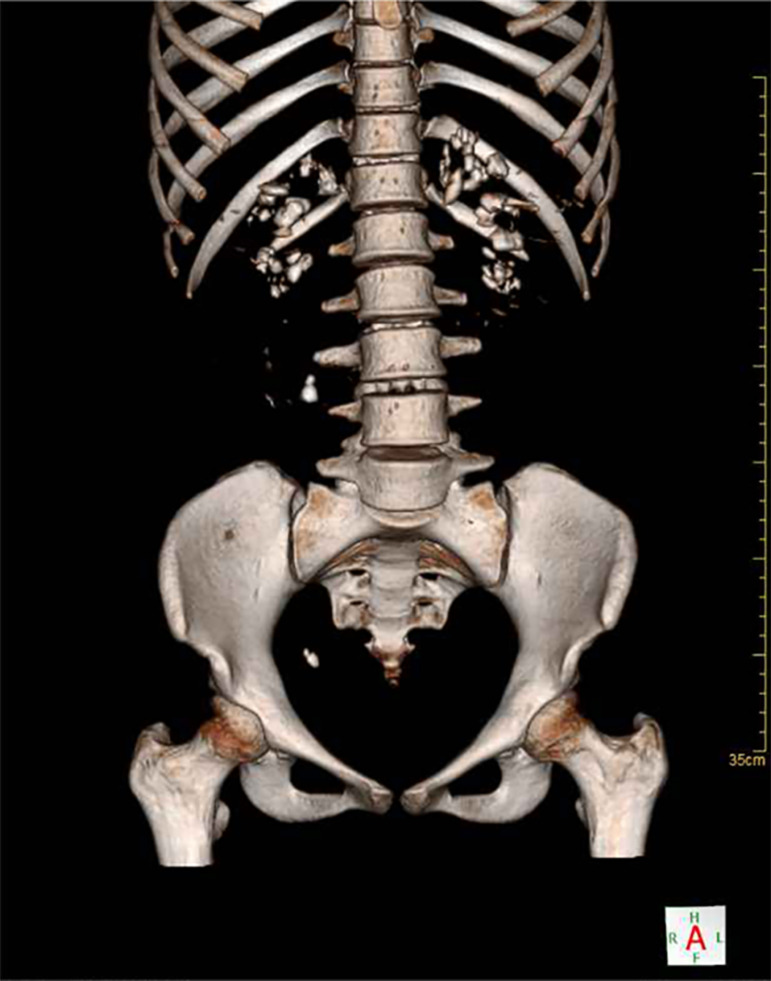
3D abdominal CT scan: bilateral nephrocalcinosis, ureteral lithiasis in the crossing of the external iliac vessels on the right, 1 cm, density 1620 uh (Hounsfield units) and ureterovesical junction.
